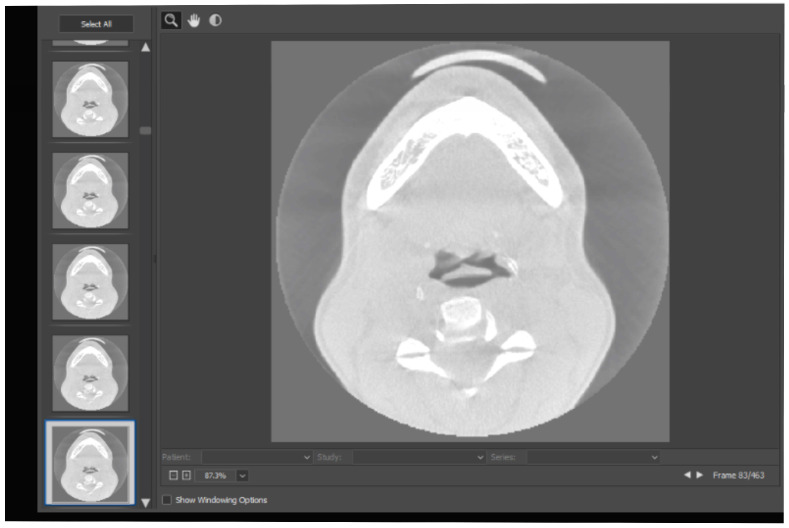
CBCT images of the analyzed patient.
